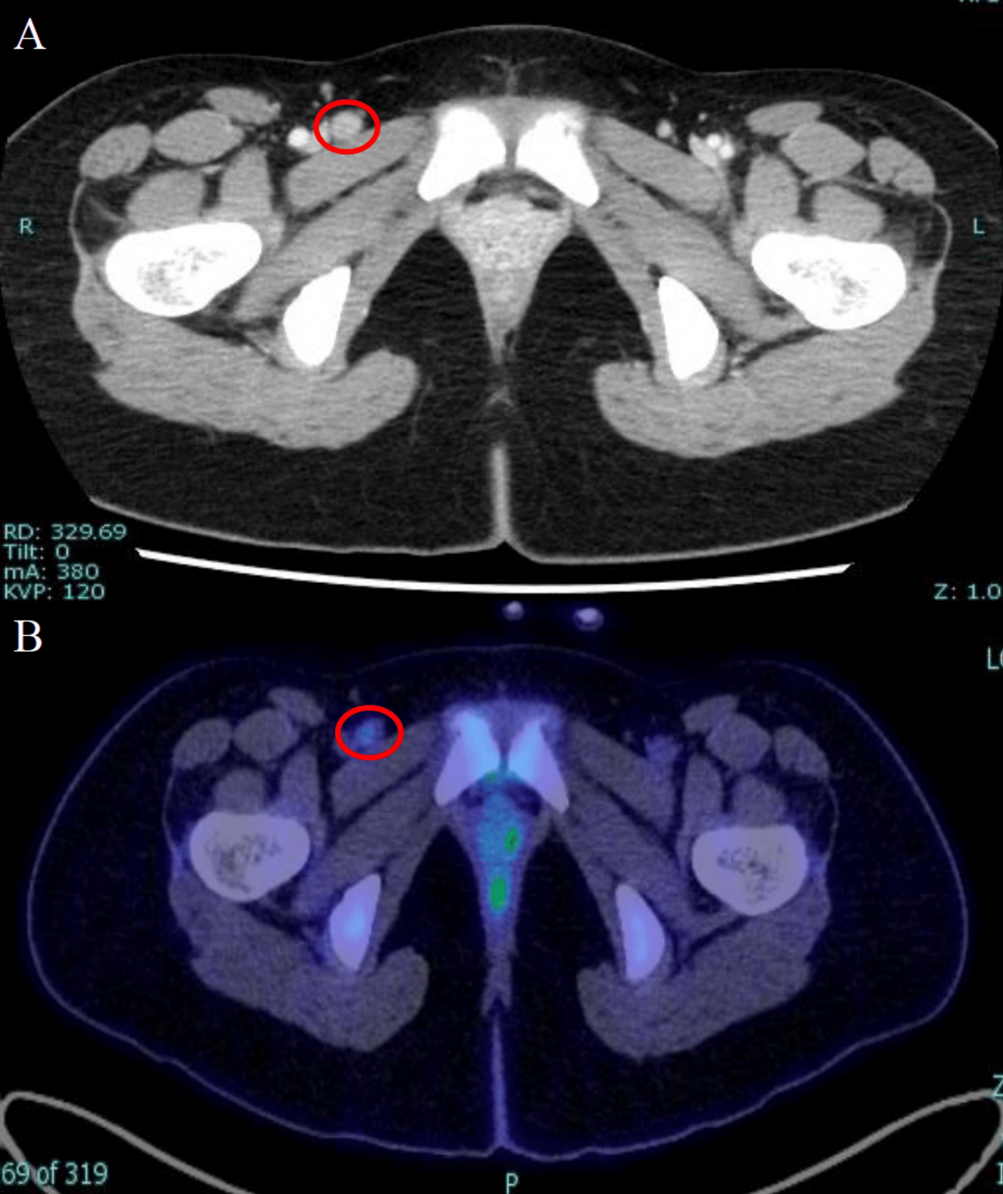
The representative images of CT and PET-CT (A) Contrast-enhanced CT shows mild enlargement of the right inguinal lymph nodes. (B) PET-CT demonstrates FDG accumulation in the same area (SUVmax 1.9). No evidence of distant metastasis is observed. CT: computed tomography; PET-CT: positron emission tomography-computed tomography; FDG: fluorodeoxyglucose; SUVmax: maximum standardized uptake value
